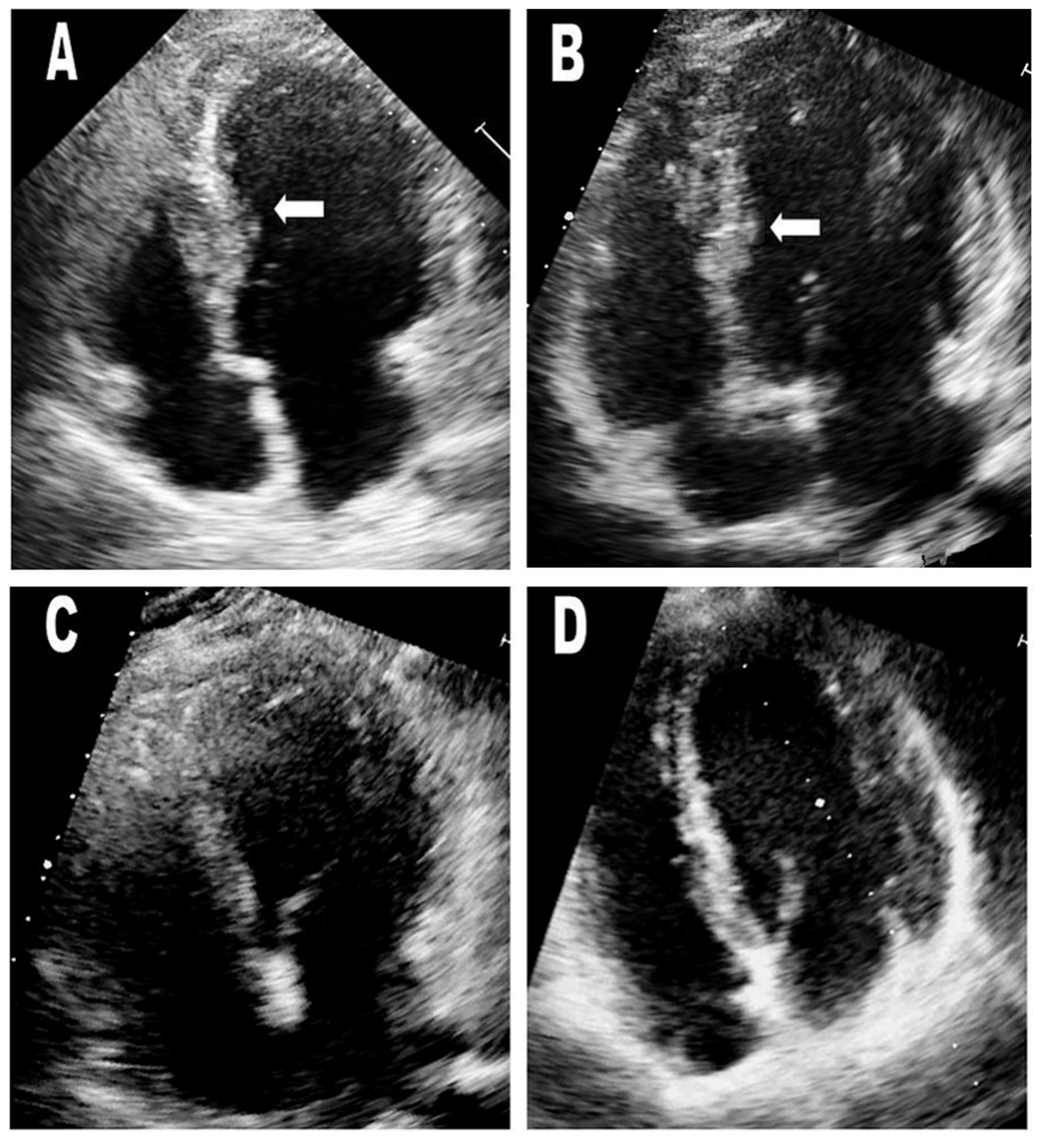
Echocardiograms of the proband (III-2) and his younger brother (III-3) (A&B). White arrows indicate areas of hypertrophy. Maximum wall thicknesses were 18 mm in the proband and 17 mm in his younger brother. Echocardiograms of heterozygous mutation carriers (I-2 and I-3) in the oldest generation were normal (C&D). White arrows indicate the interventricular septum.
